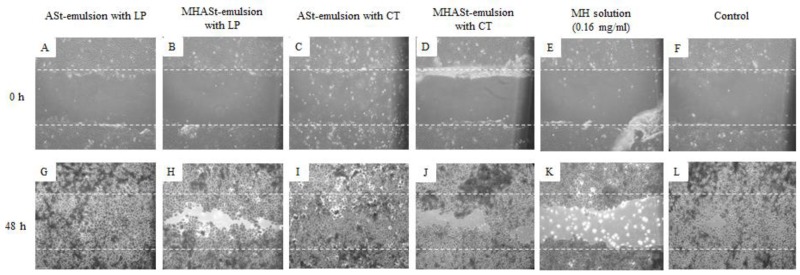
Representative time-lapse images of HaCaT keratinocyte scratch assays immediately after the scratches had been made and then after 48 h in the presence of 5%-emulsions with LP (A,G), 5%-MHASt-emulsions with LP (B,H), 5%-ASt-emulsions with CT (C,I), 5%-MHASt-emulsions with CT (D,J), MH solution (E,K) or control medium (F,L). The cells were allowed to migrate for 48 h, fixed and photographed. Outlines of the original wounds are marked with dashed lines. Original magnification 40×.
